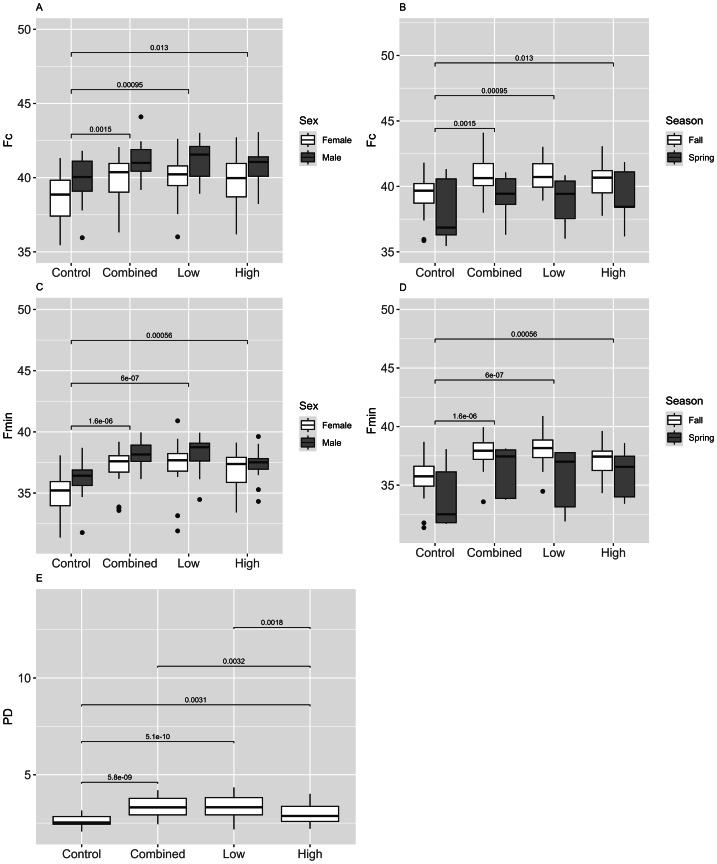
Differences in echolocation characteristics of evening bats (*Nycticeius humeralis*) during ultrasonic deterrent trials. Asterisks indicate significant differences (* = 0.05,  = 0.01, * = 0.001, and  = 0.0001). Treatments included Control and ultrasonic deterrent emissions (Low (20–32 kHz), High (38–50 kHz), and Combined (20–50 kHz)). (A) characteristic frequency (Fc), (B) maximum frequency (Fmax), (C) minimum frequency (Fmin), (D) difference between Fmax and Fmin (Fdiff), and (E) pulse duration (PD). Significant differences are indicated above the box plots.
